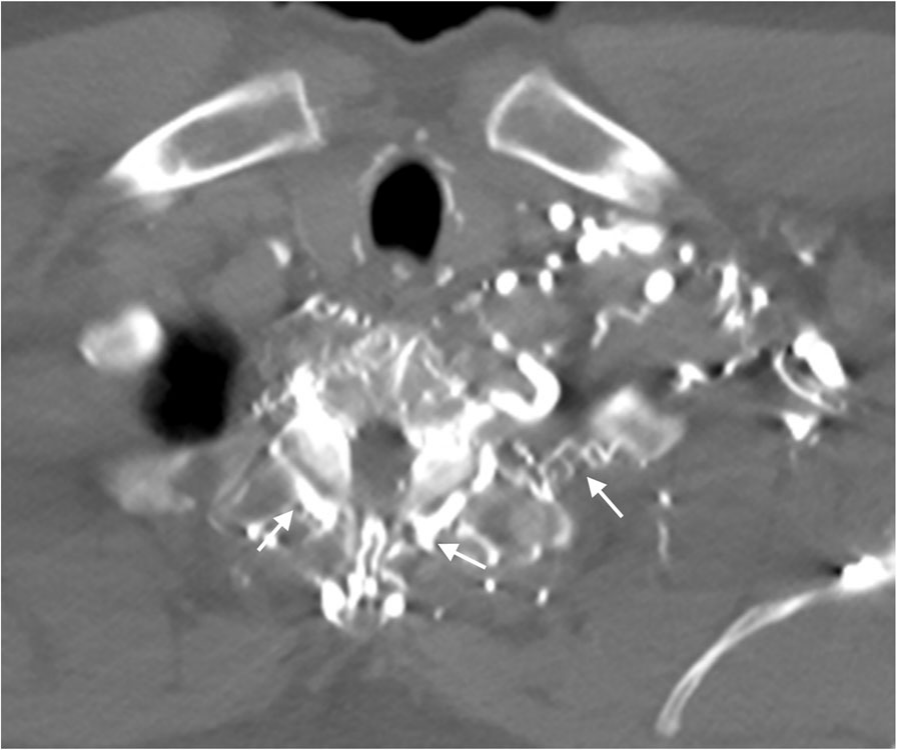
Posterior neck collaterals. Axial CT image showing extensive unnamed vertebral collaterals (arrows) in a patient with occlusion of the left brachiocephalic vein
